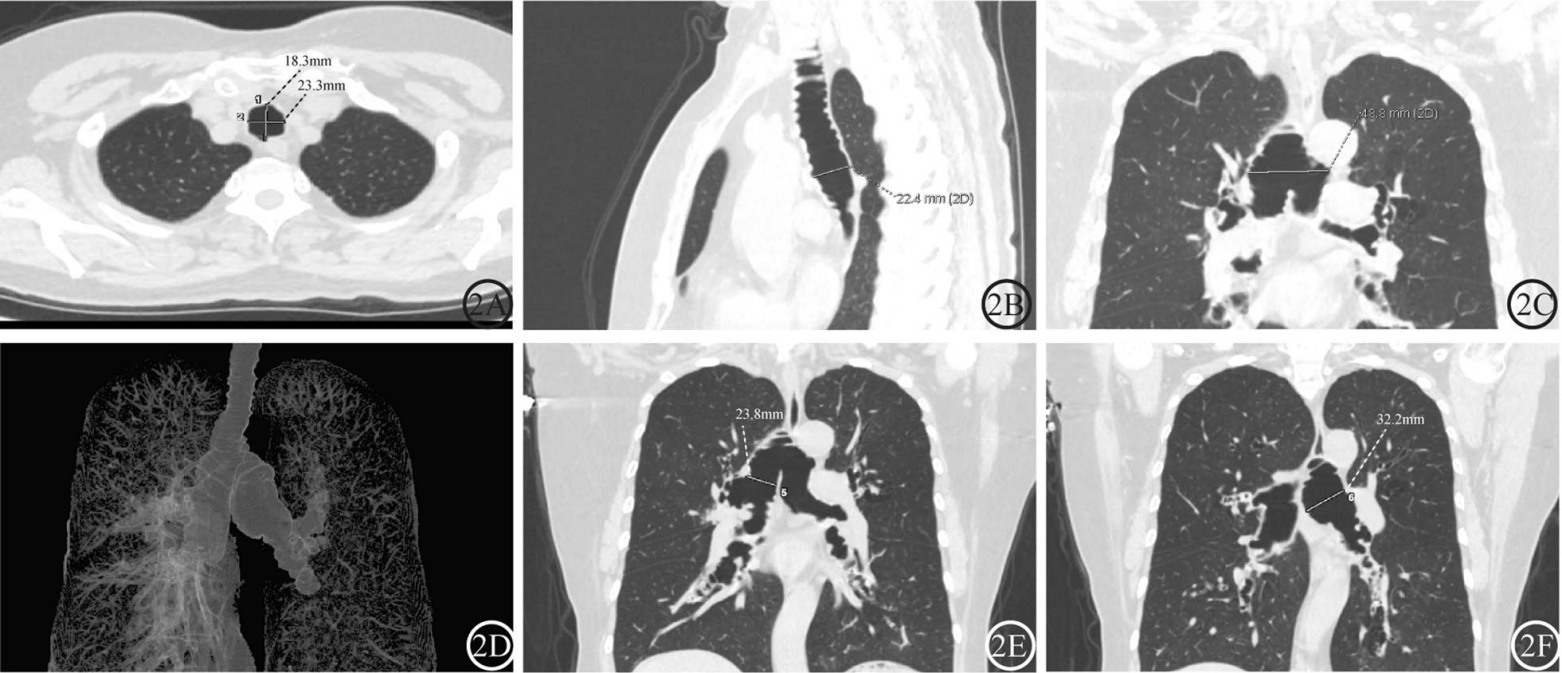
Multimodal CT evaluation of MKS (Case 4). A–C Axial, sagittal, and coronal views demonstrating pantracheobronchial dilation. D 3D airway reconstruction reveals main bronchial dilatation with pathognomonic features: multiple diverticula and cystic bronchiectasis. E, F Coronal oblique MIP images confirming bilateral mainstem bronchus dilation. Abbreviations: MKS: Mounier–Kuhn syndrome; MIP, maximum intensity projection; CT, computed tomography
